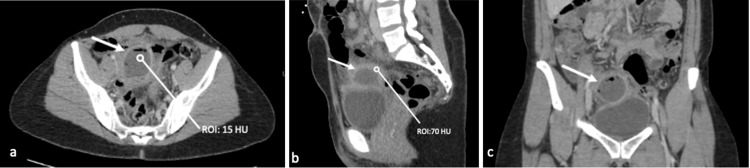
CT abdomen pelvis with IV contrast CT abdomen and pelvis with contrast axial (a), sagittal (b), and coronal (c) images demonstrate a thick-walled fluid-like collection (white arrow) containing gas superior to the bladder with an internal density of 15 HU and rim density of 70 HU.
